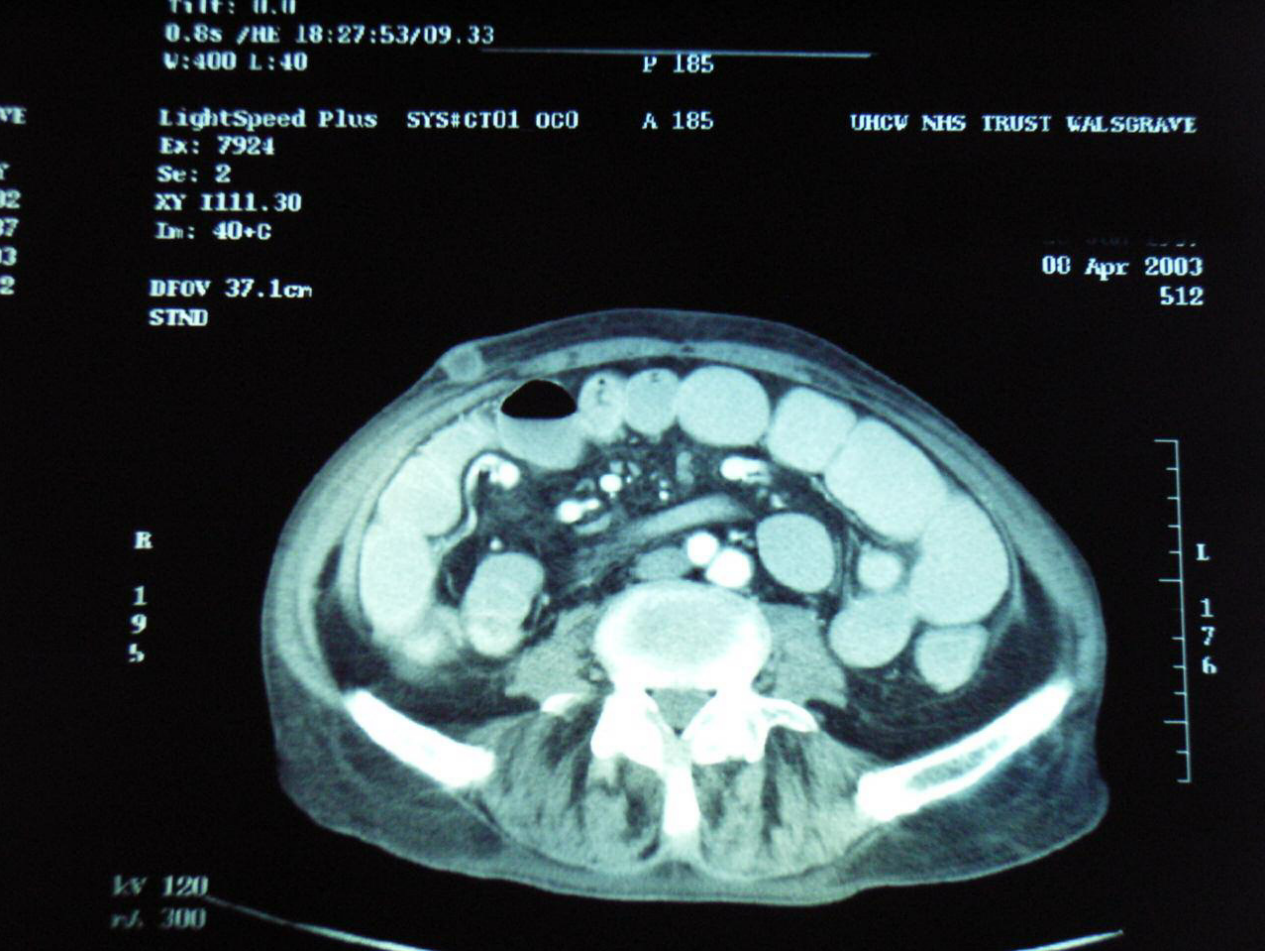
Abdominal CT section showing a subcutaneous nodule on the right abdominal wall.
